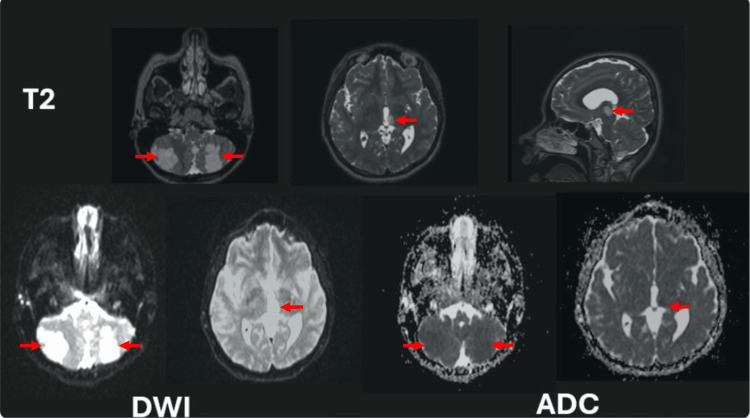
Brain magnetic resonance angiography (axial and sagittal T2, axial DWI, and ADC sequences). Multiple infarctions are observed in the left thalamus and both cerebellar hemispheres (red arrows), corresponding to the vascular territories of the left posterior cerebral artery (PCA) and the bilateral posterior inferior cerebellar arteries (PICA). DWI, diffusion-weighted imaging; ADC, apparent diffusion coefficient
